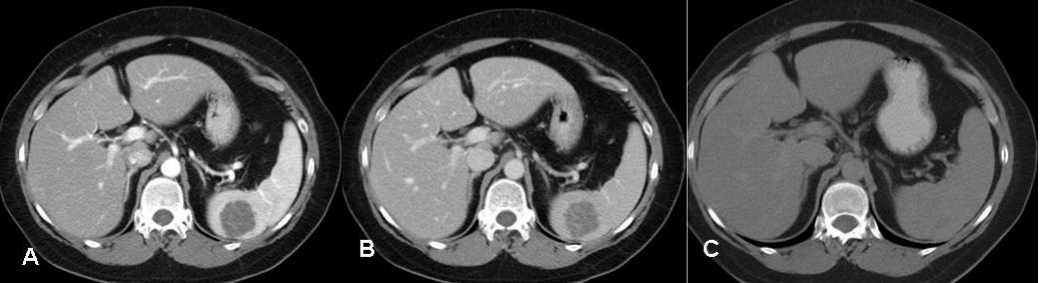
CT scan after oral and iv-contrast in the arterial phase A and portal venous phase B demonstrates a hypodense well defined round lesion in the posterior portion of the spleen with some linear internal enhancement. C. The lesion is isodense compared to the normal spleen on the 5 minutes delayed image.
